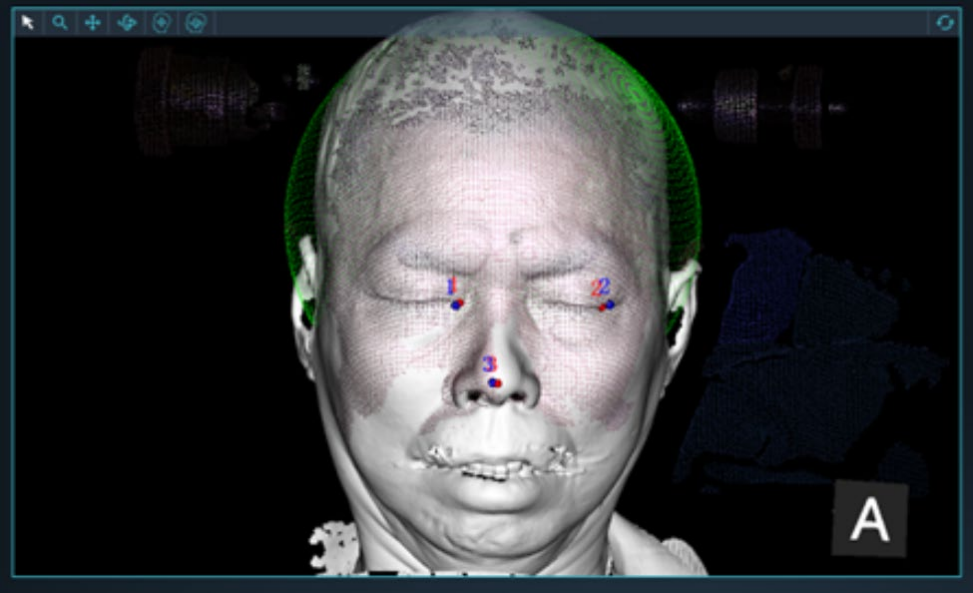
Matching of slected points between the 3D Sstructured light scanning extraction and CT 3D reconstruction
